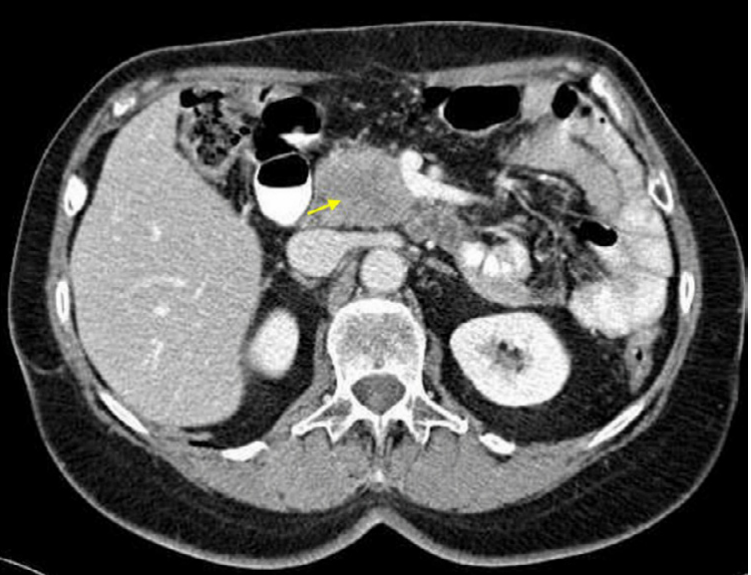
Computed tomography of the abdomen four years after Billroth II resection for gastric cancer, revealing an inhomogenous mass in the pancreatic head, 4 cm in diameter. (Picture courtesy the Division of Radiology, German Cancer Research Center, provided by PD Dr. med. S. Delorme).
